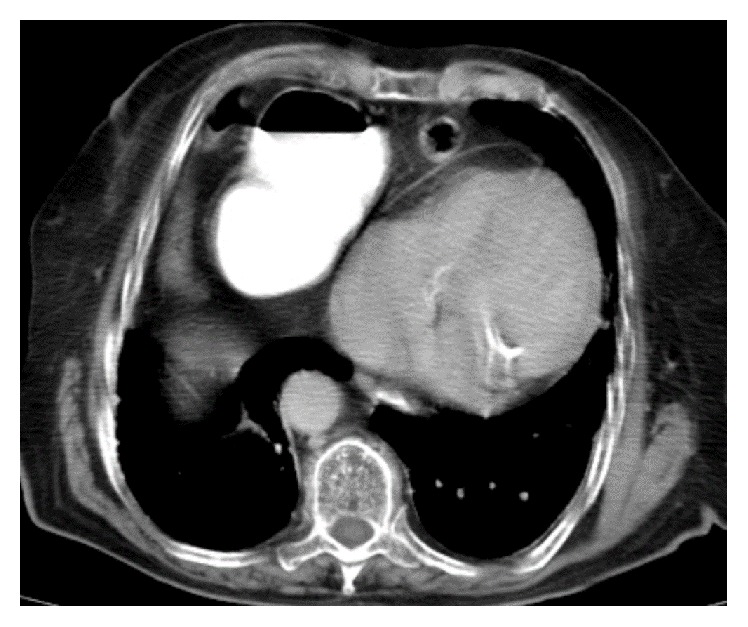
Thoracic CT scan. Stomach filled by contrast in right hemithorax.
